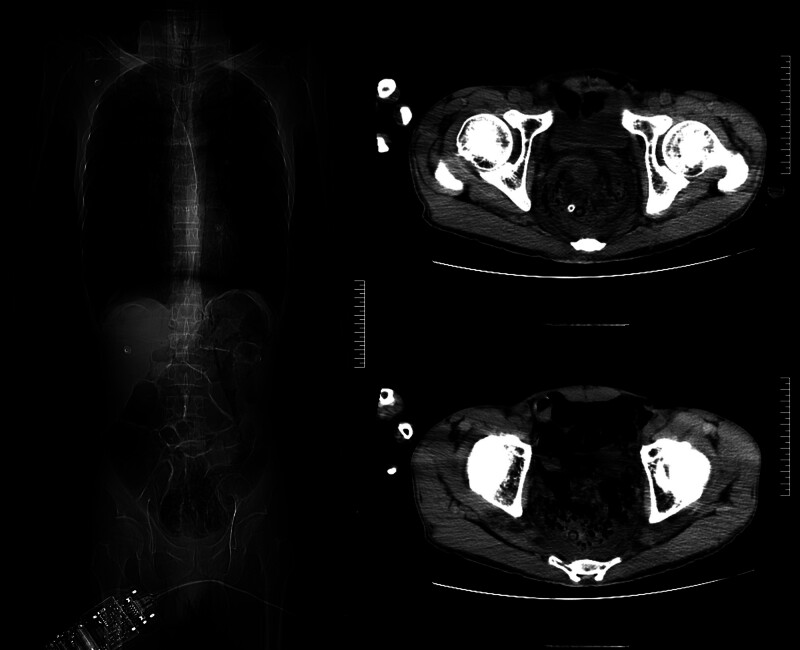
Computed tomography (CT) on January 05, 2024.
